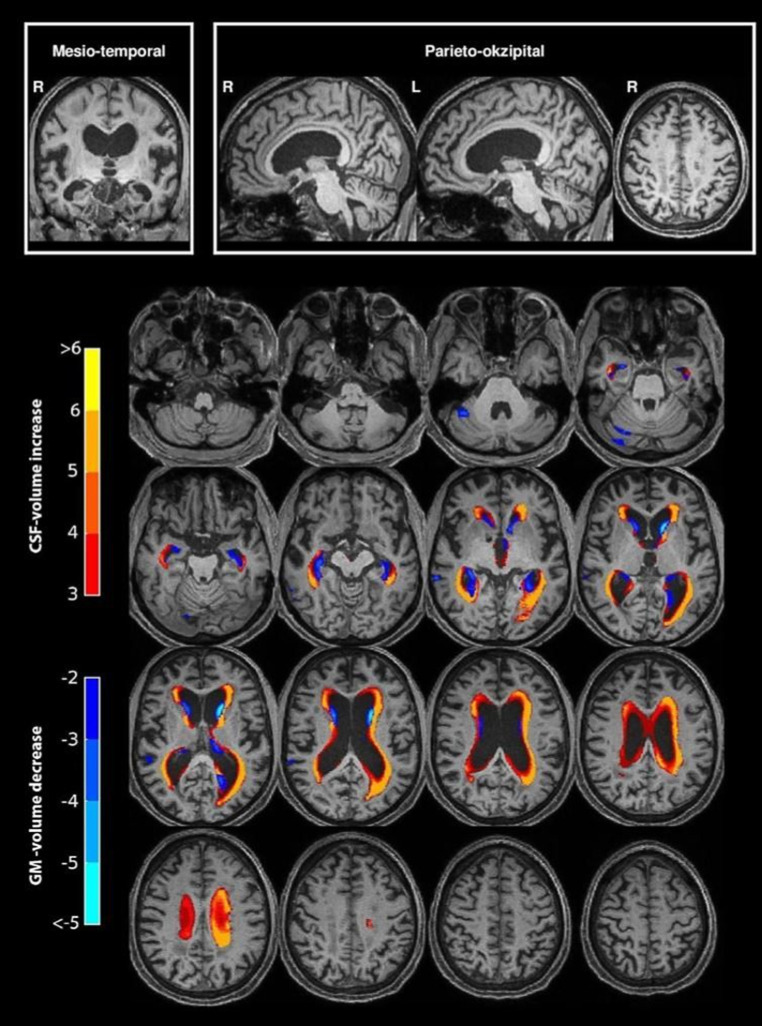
Support vector machine (SVM) results showing abnormal gray matter (GM) and cerebrospinal fluid (CSF) regions superimposed in color on a normal pressure hydrocephalus (NPH) patient’s (82, male) individual 3D T1-weighted brain MRI in serial sections from caudal to cranial (increase of CSF volume in *red to yellow*, and decrease of GM volume in *dark to light blue*). On the left color bars indicate the corresponding z‑scores. SVM analysis came to a prediction score of 0.94. Additionally, representative brain slices (from *left* to *right*: coronary, parasagittal right and left, axial close to the convexity) are presented in the top row with a typical disproportionately enlarged subarachnoidal space hydrocephalus (DESH) sign in this specific NPH case (*top left*)
